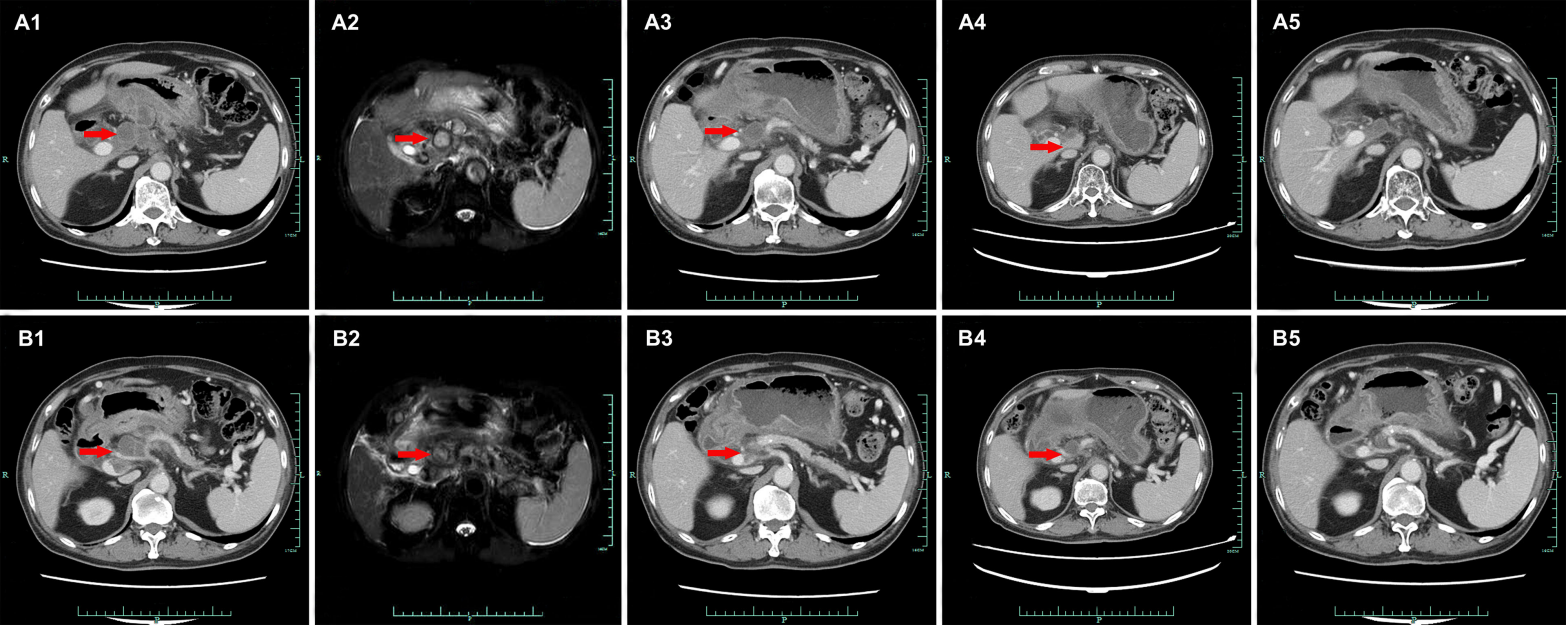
Abdominal CT presentations after recurrence (from July 11, 2020 to August 4, 2021, red arrows for tumors). (A1/B1) July 11, 2020. (A2/B2) October 22, 2020. (A3/B3) January 25, 2021. (A4/B4) April 6, 2021. (A5/B5) August 4, 2021.
